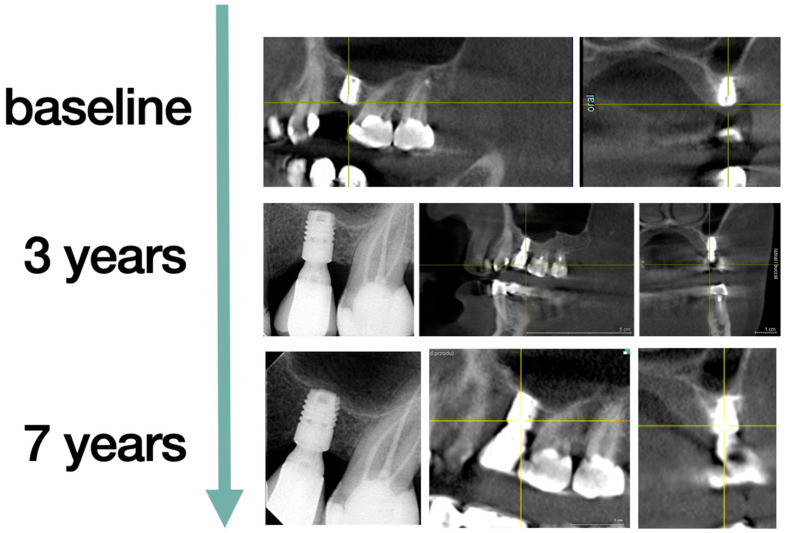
Short implants. The same patient from baseline to seven years, implant tooth 26, follow-up. Periapical X-ray and Cone Beam Computed Tomography (CBCT) are presented.
